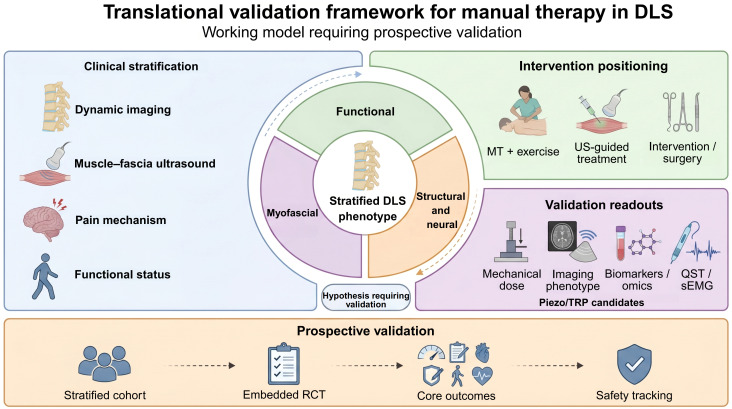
Prospective validation framework for the manual therapy-related mechanotransduction hypothesis in DLS. The left panel shows that clinical stratification should integrate dynamic imaging, muscle-fascia ultrasound, pain mechanisms, and functional status to identify function-dominant, myofascial-dominant, and structural/neural-dominant DLS phenotypes. The upper-right panel shows phenotype-specific intervention positioning, including manual therapy plus exercise, ultrasound-guided treatment, and interventional or surgical escalation. The lower-right panel shows validation readouts, including mechanical dose, imaging phenotype, biomarkers/omics, QST/sEMG, and Piezo/TRP candidate indicators. The bottom band proposes a prospective validation pathway, including stratified cohorts, embedded randomized controlled trials, core outcome assessment, and safety tracking, to provide a structured research framework for the manual therapy-related mechanotransduction hypothesis. Created in BioRender. HA, Y. (2026) https://BioRender.com/6vxsgc0.
